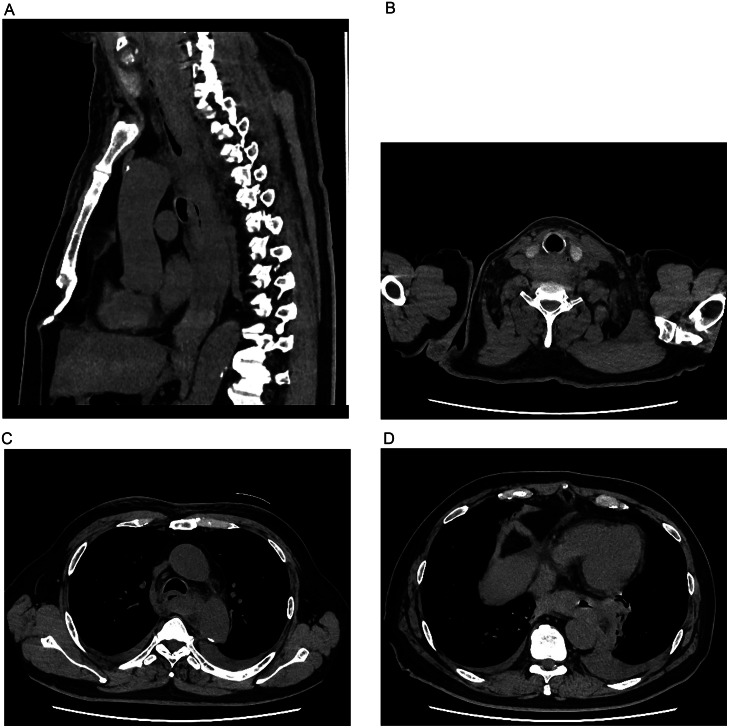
(A) Precontrast CT scans in the sagittal plane (A) and axial plane (B-D) depict an extensive hematoma extending from the neck to the mediastinum, surrounding the esophagus (C, D).
